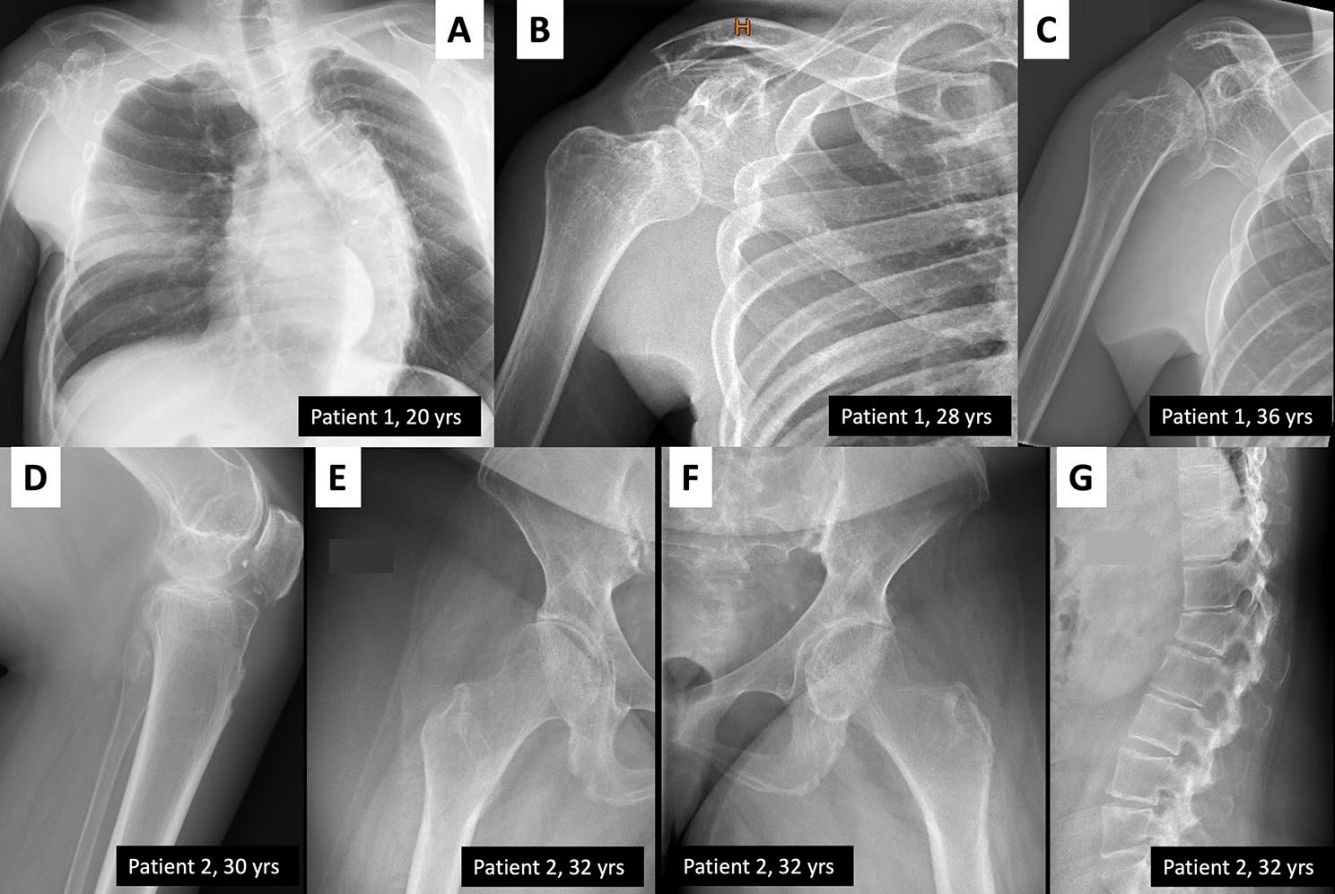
Progression of skeletal changes during adulthood in patients of Family 2. (A, B, C) Radiographs of Patient 1 at ages 20, 28 and 36 years, showing severe scoliosis and progressive deformity and osteolysis of the proximal humerus. (D, E, F, G) Radiographs of Patient 2 at ages 30 and 32 years show generalized osteopenia, osteolysis and osteoarthritis in the knee and hip joints, and a vertebral compression fracture in the spine
